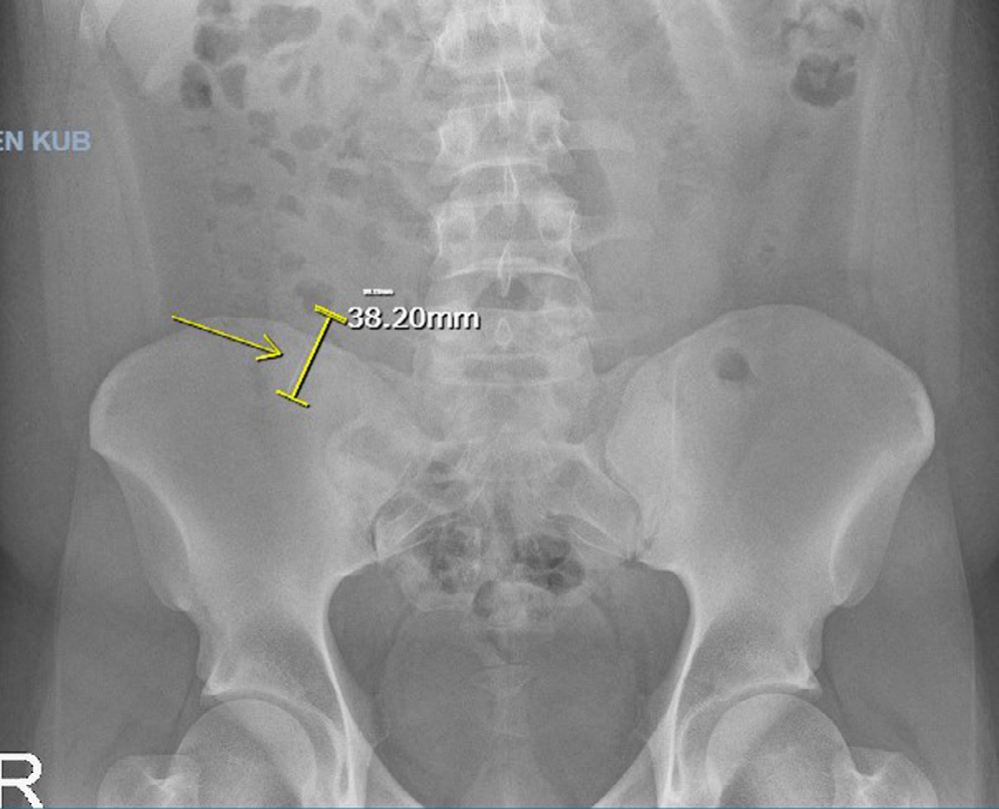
X-ray of abdomen demonstrating partial small bowel obstruction. A repeat X-ray of the kidneys, ureters and bladder revealed mildly dilated small bowel loops representative of ileus or partial small bowel obstruction. Arrow indicates a radiopaque foreign body in the right lower abdomen without evidence of mass effect. There is no evidence of free air. Measurement approximately 38 mm.
